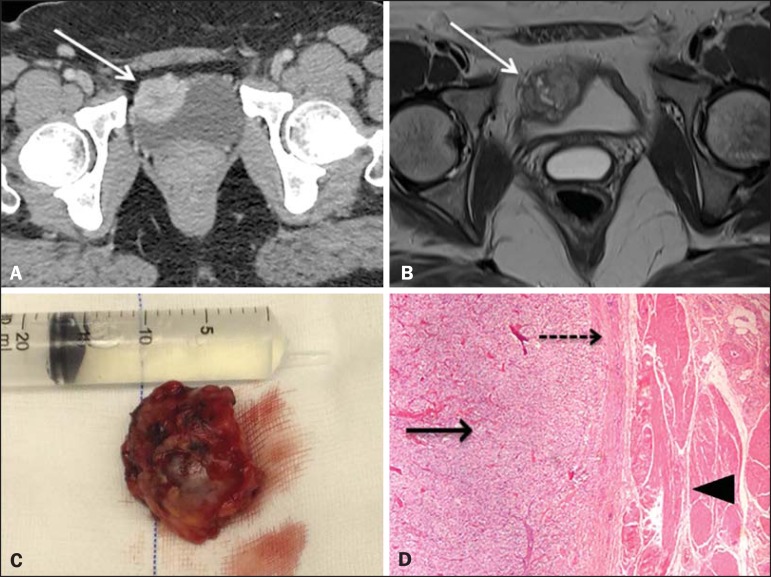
A: Intravenous contrastenhanced axial CT scan showing a
